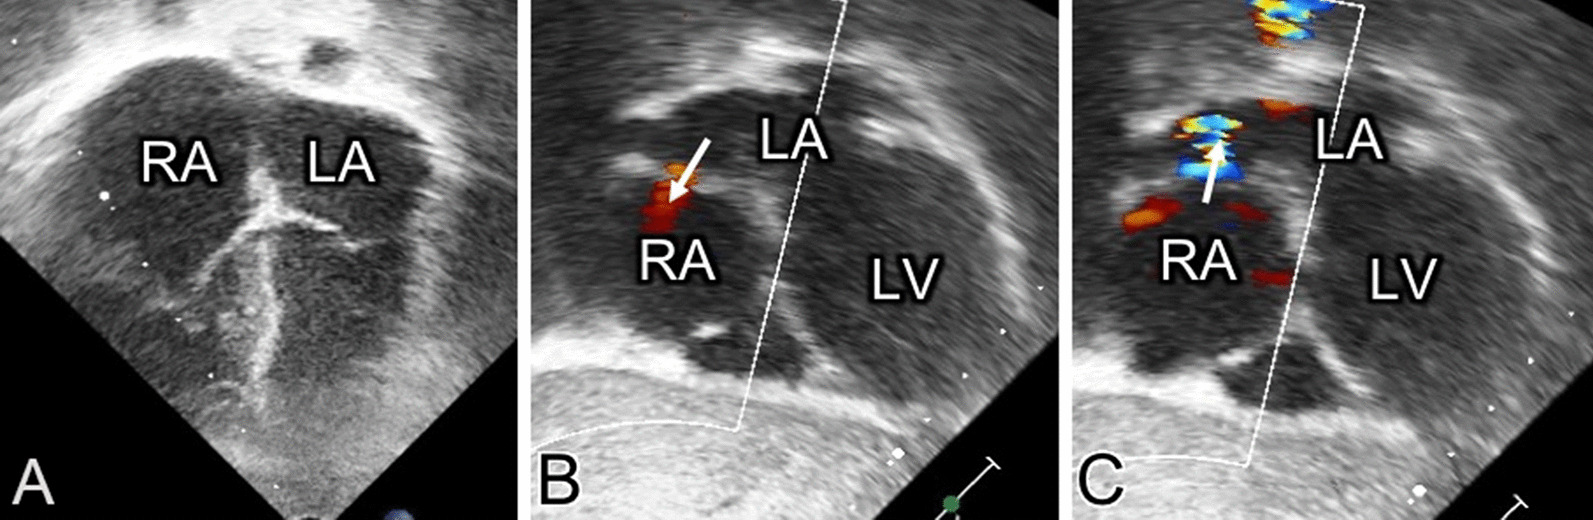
Postsurgical echocardiography. A shows an apical-4-chamber plane with convex interatrial septum. B, C show a subcostal 4-chamber plane. The arrow points the bidirectional shunt in foramen oval. *RA: Right atrium; LA: Left atrium: LV: Left ventricle*
